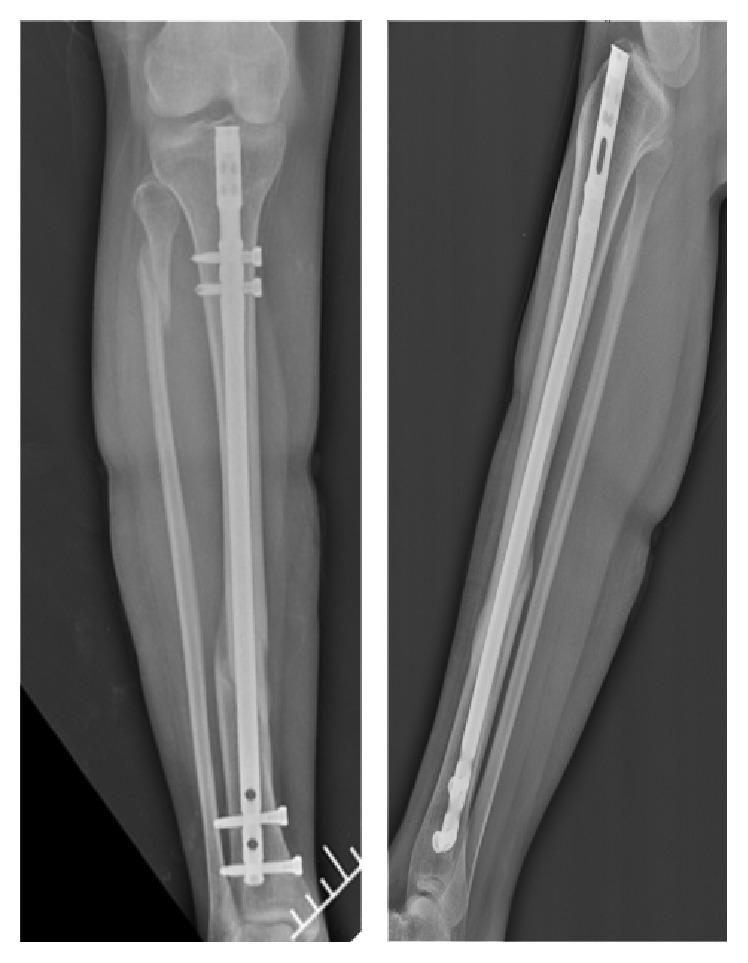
Radiographs showing united tibia at four months.
